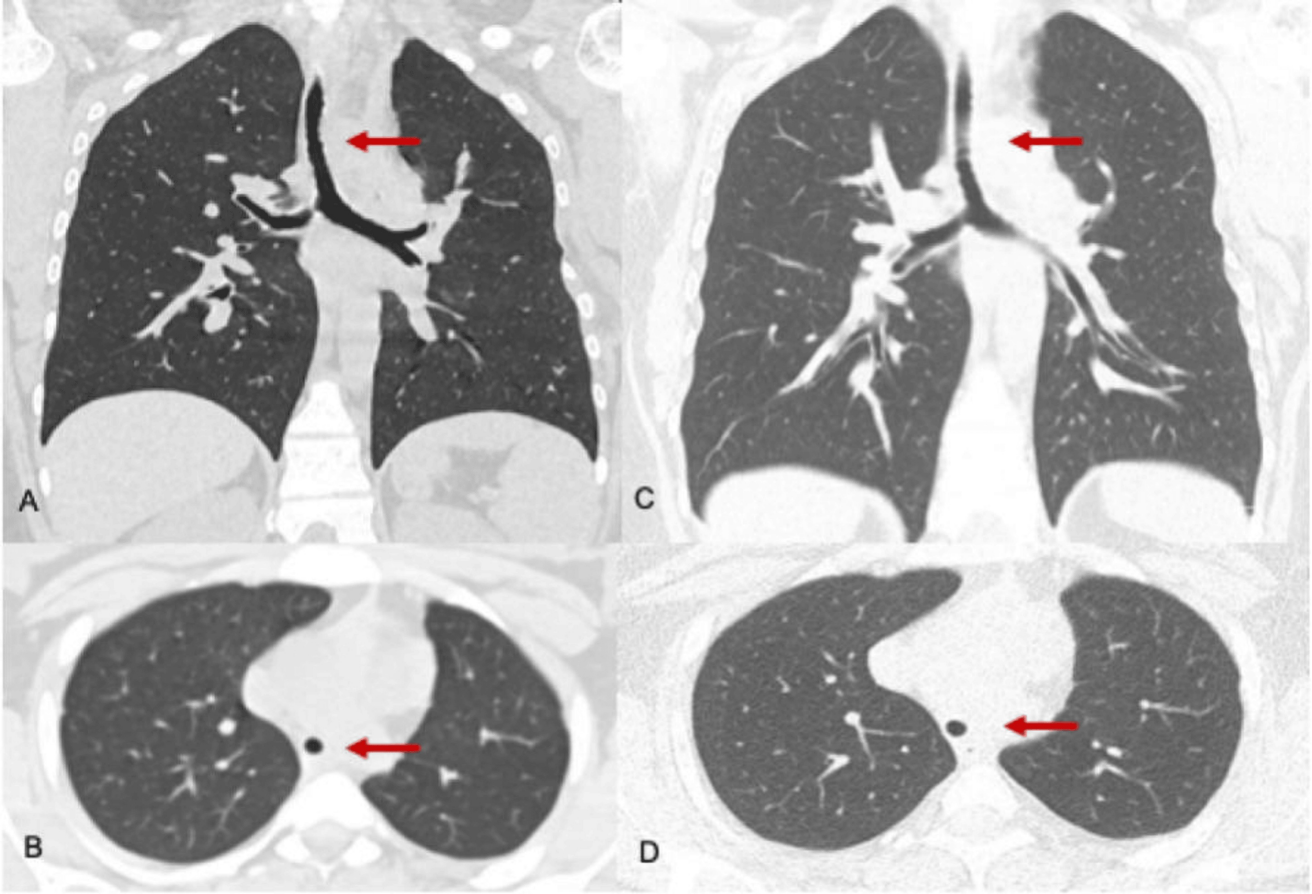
Coronal and sagittal CT scans of the chest demonstrating stable diffuse tracheal obstruction at 13 (A, B) and 19 (C, D) years of age. The images show persistent diffuse narrowing of the thoracic trachea, with a minimum luminal diameter of 6-7 mm.
